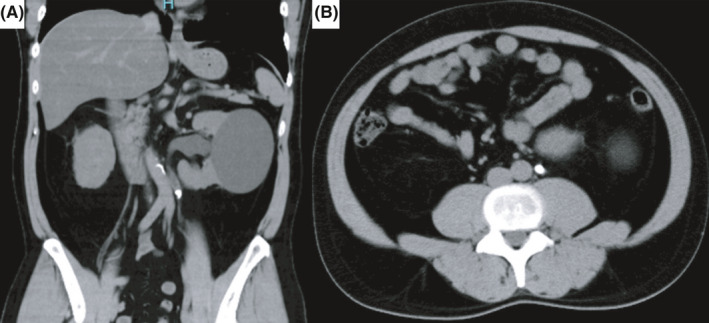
A, Coronal and (B) axial CT images of initial ureteral stone
